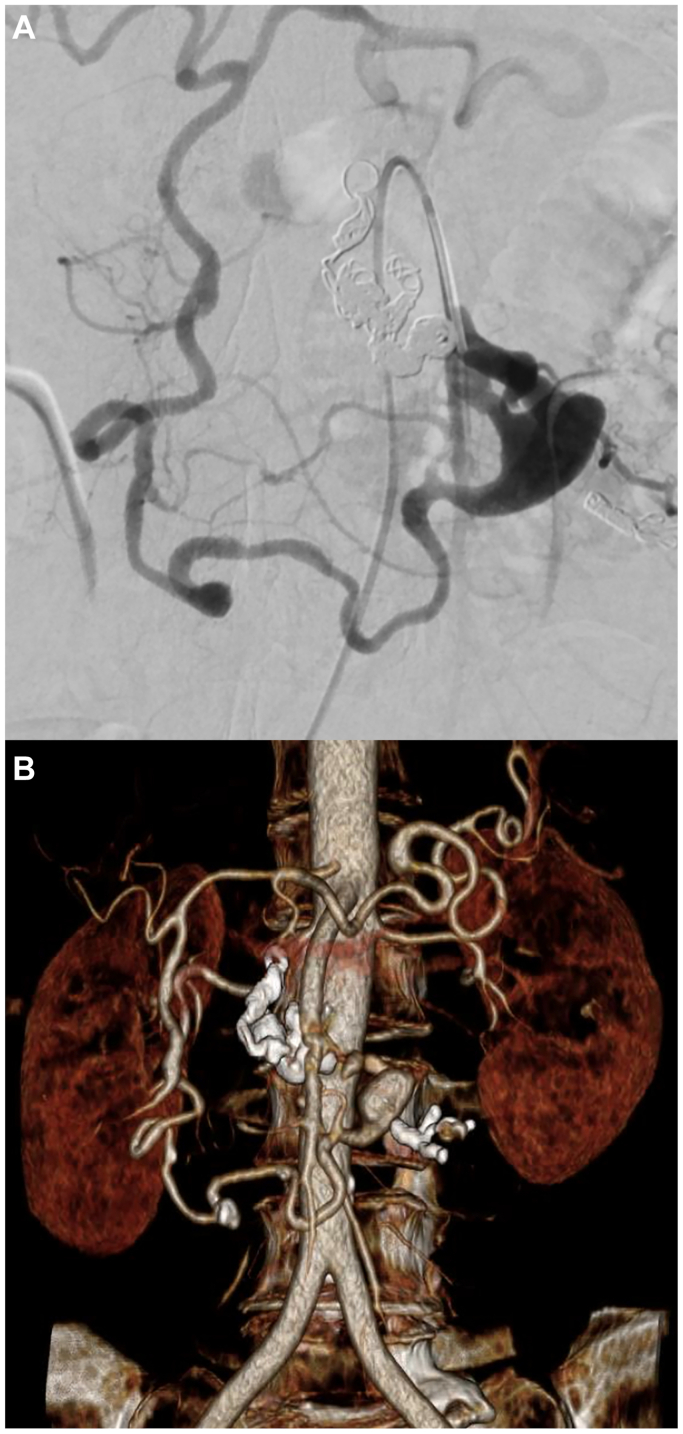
(A) Postembolization digital subtraction angiography shows the subtracted cast of coils after packing of the arc of Bühler (AOB), exclusion of the pseudoaneurysm and preserved patency of the celiac-mesenteric collateral circulation. (B) Volume reconstruction of the 7-day follow-up computed tomography angiography confirming the postembolization outcome, with maintained patency of the celiac-mesenteric circulation.
